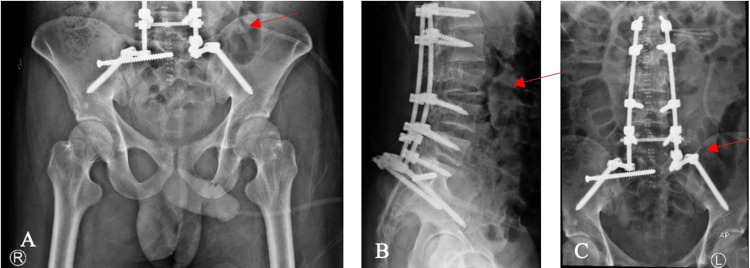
Post fixation X-rays A: Anteroposterior (AP) view of the pelvis; B: Lateral; C: AP view of fixation. The arrows show the fixation.
